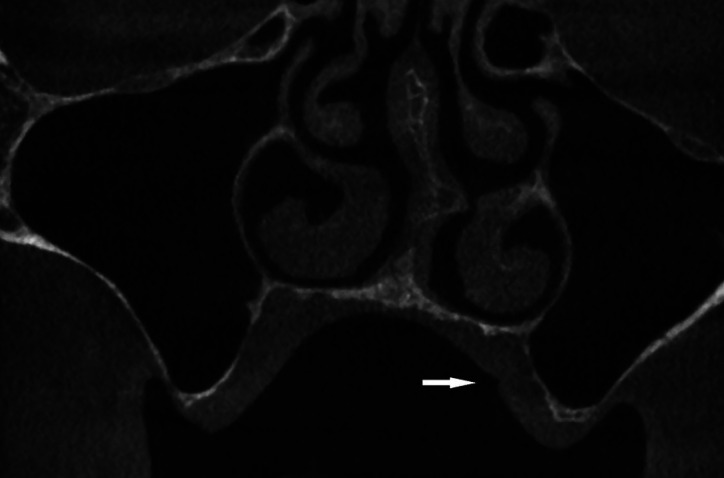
The cone beam computed tomography examination of the lesion area (white arrow). No bony pathology was found.
